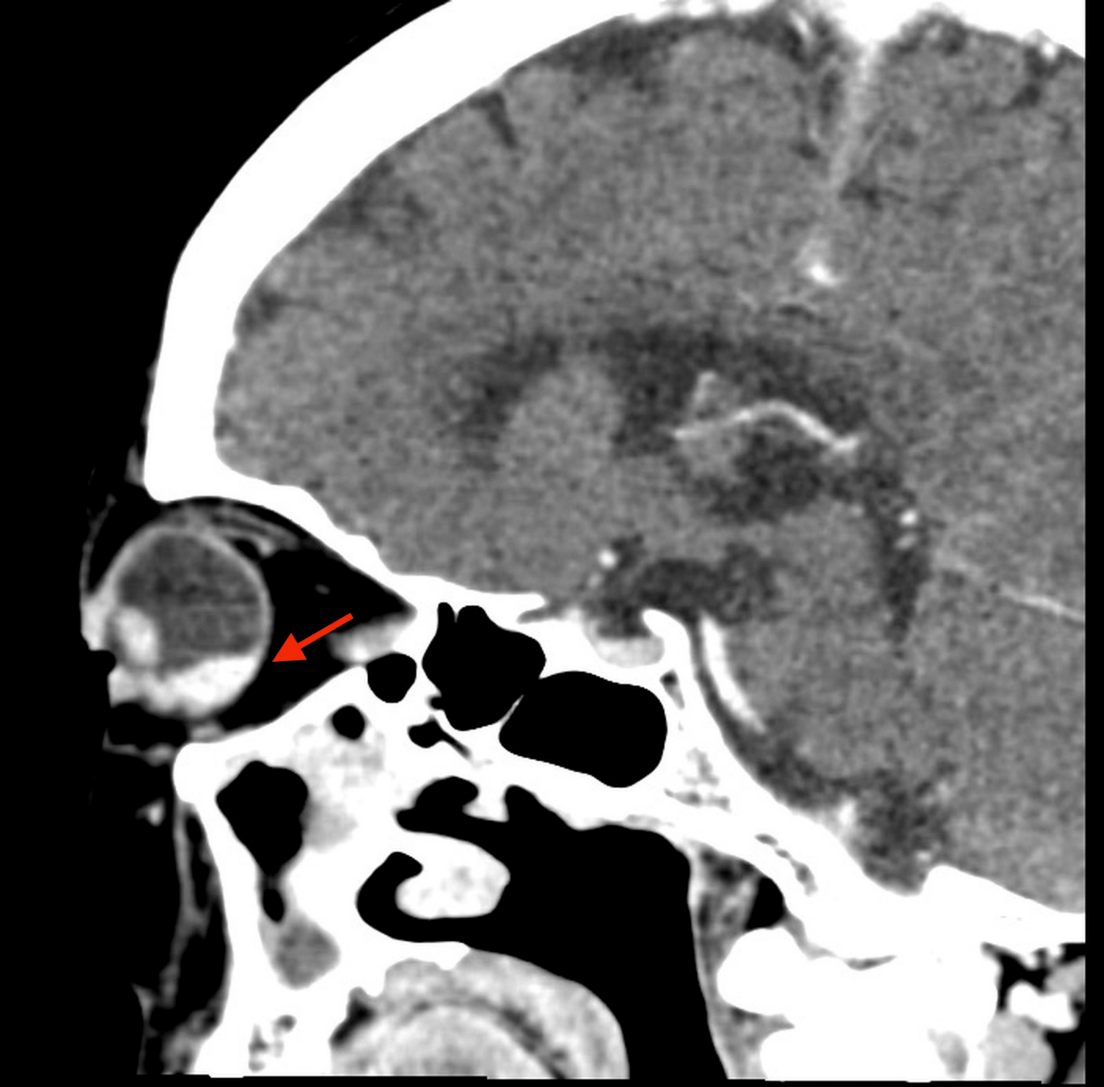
Contrast-enhanced CT showing a left choroidal lesion (red arrow).
